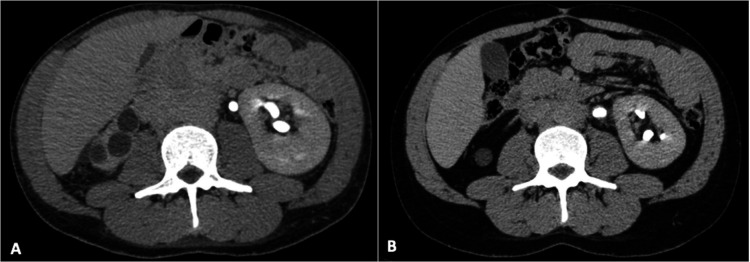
Abdominal CT axial plane: A – Initial examination on admission. Note large mass involving the abdominal aorta; B – CT scan performed 7 months after the first examination and under treatment with corticosteroid. Note the expressive reduction of the periaortic mass.
